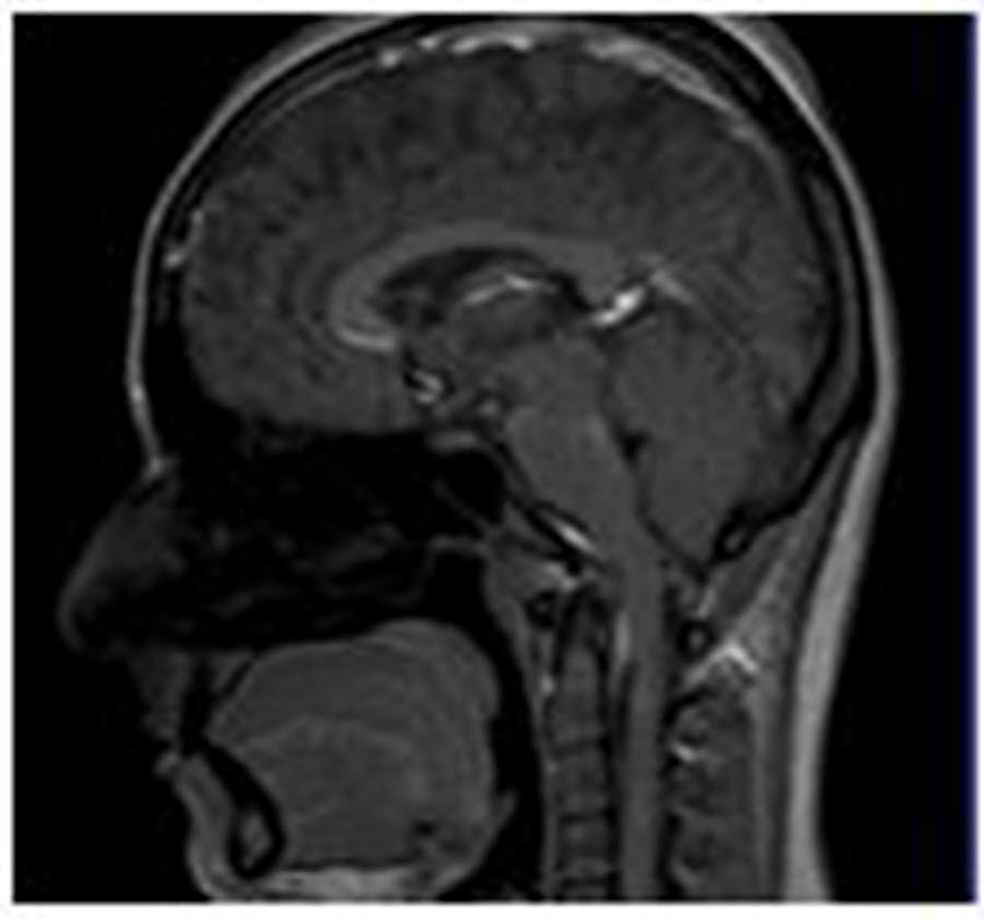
Sagittal view of TMJ using the MRI imaging modality TMJ: temporomandibular joint
